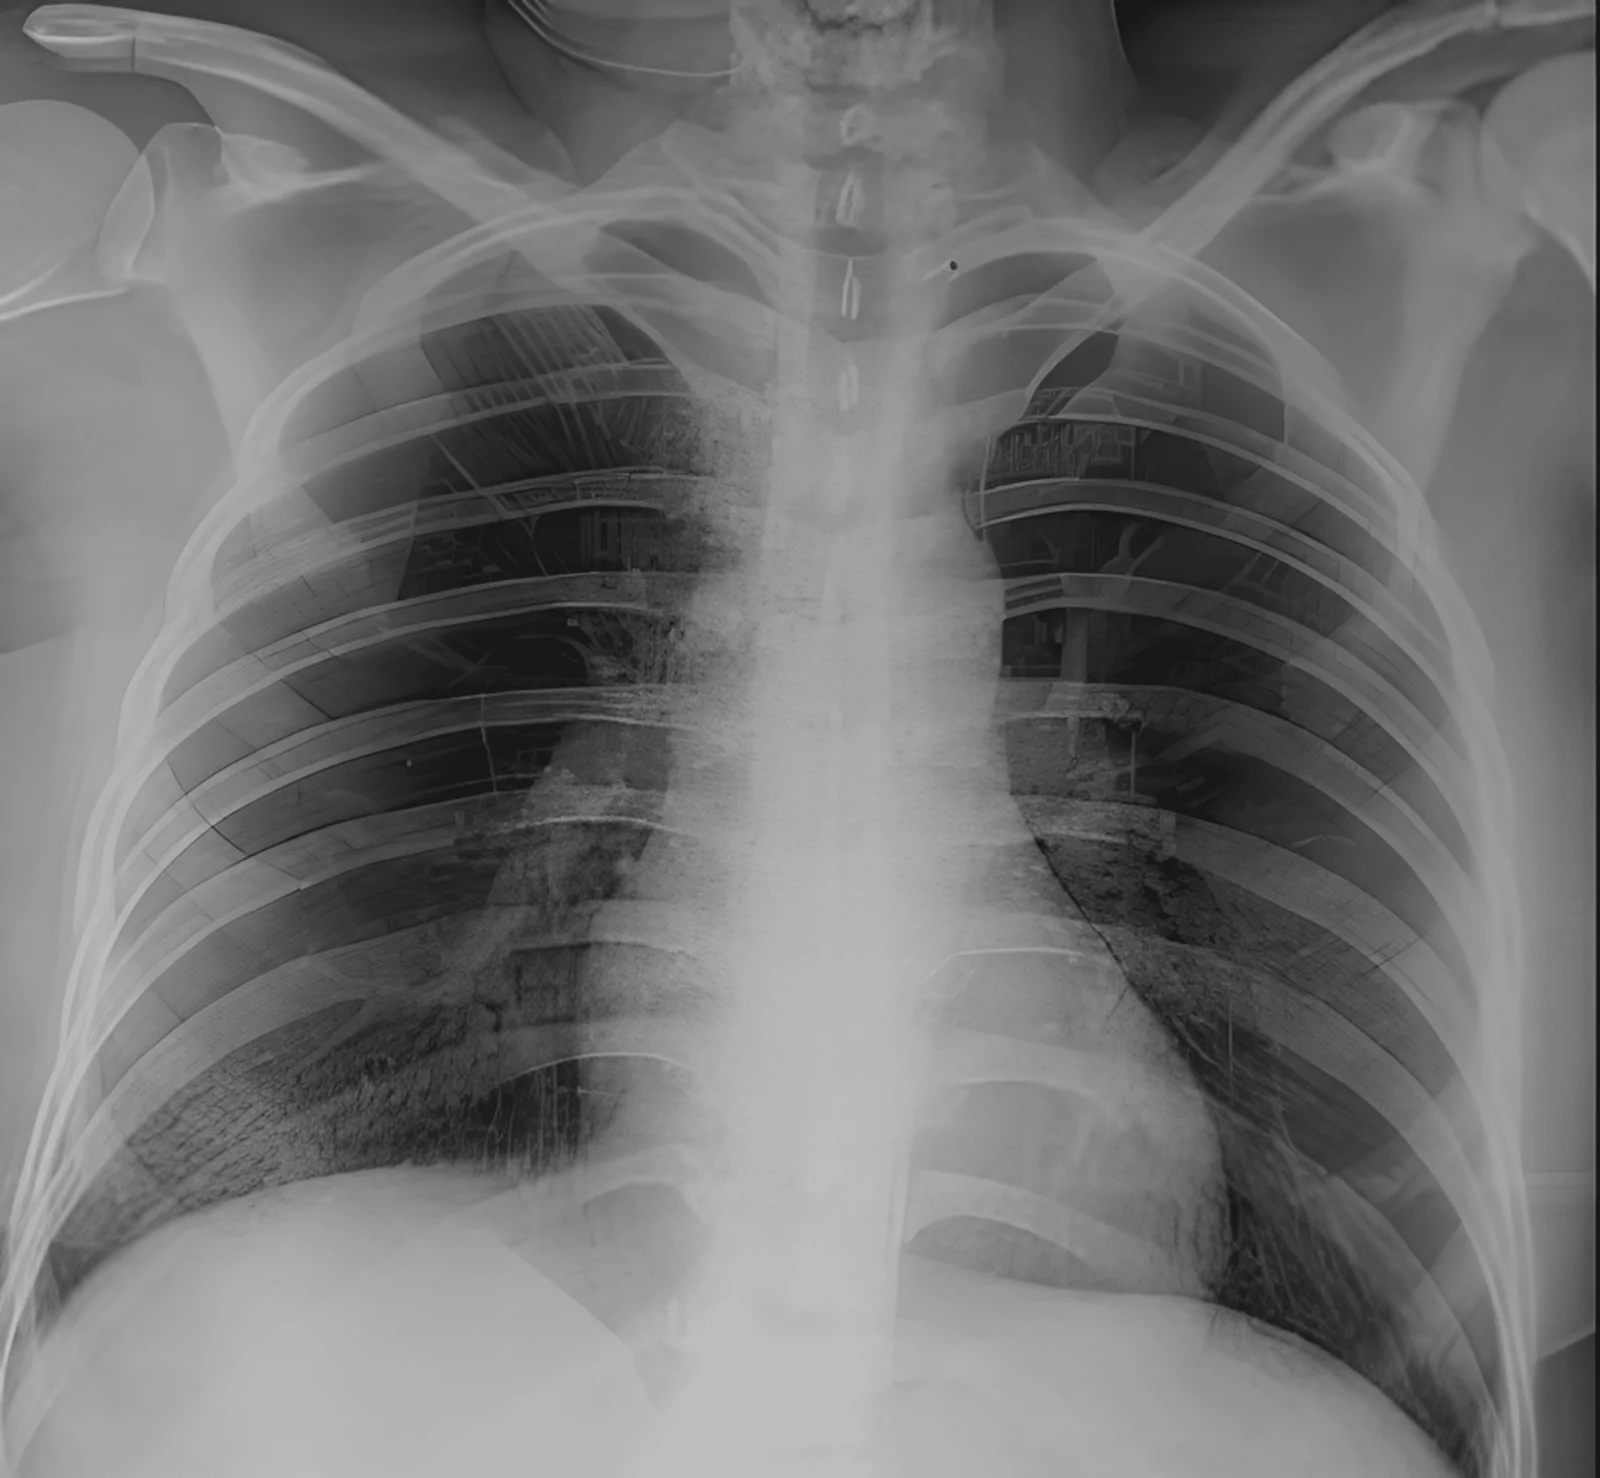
Normal Chest X-ray Chest radiograph revealed no focal pulmonary infiltrates, pleural effusion, or other acute cardiopulmonary abnormalities
